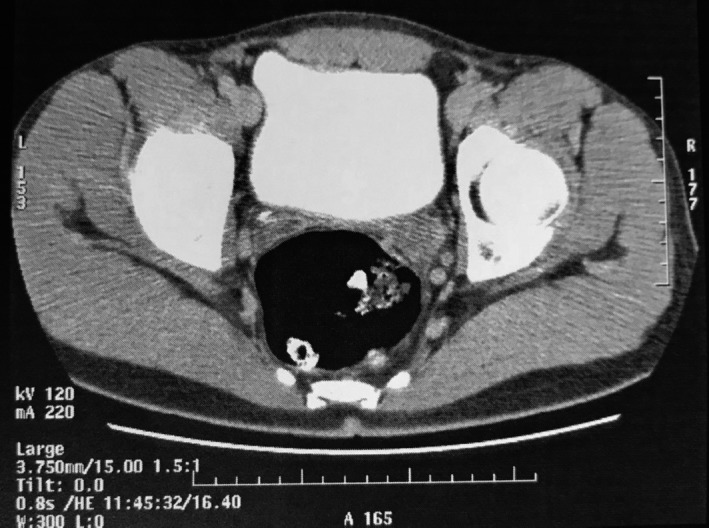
Axial view of first CT Urography (CTU), without any intravesical papillary mass or indication of filling defect
